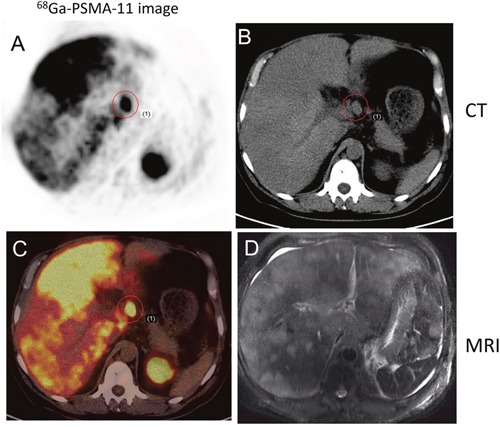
68Ga-PSMA-11 PET/CT versus T2WI MRI in a 52-year-old patient with progressive disease PCa was confirmed by lymph node aspiration; the serum PSA level was >10,000 ng/ml at the time of imaging. ^68^Ga-PSMA-11 PET/CT detected multiple lymph node and liver metastases. A. Transverse image. B. CT. C. Multiple sites of focal uptake in the liver. D. MRI.
